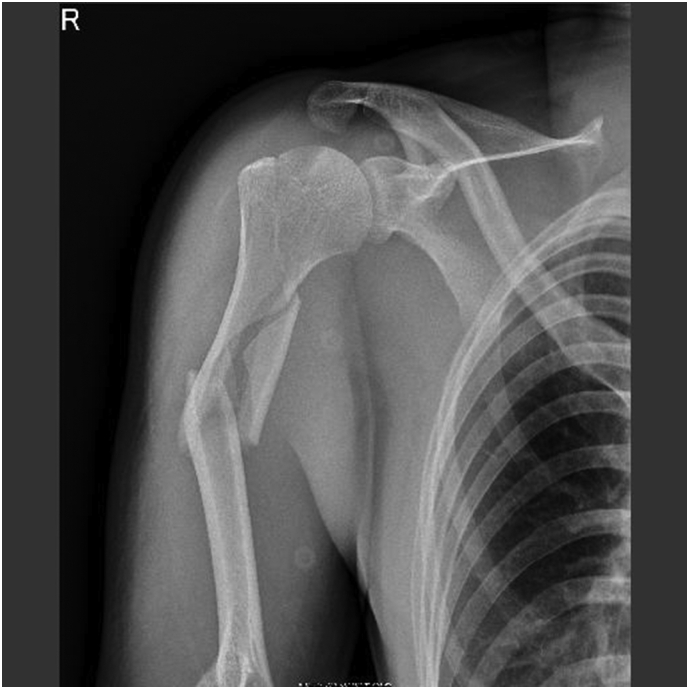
X-ray of after closed reduction.
